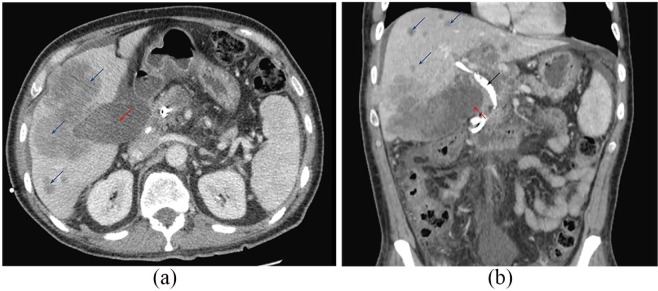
(a) CT scan at 3 week after admission. Gallbladder lesion (red arrow). There
